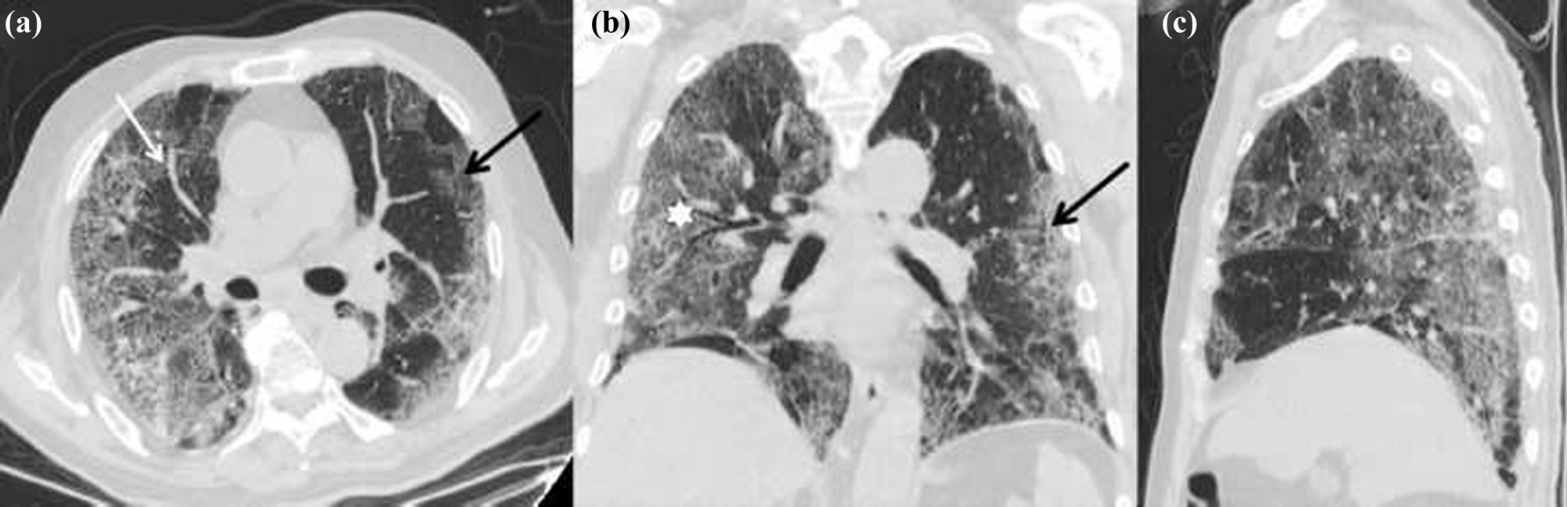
First CT scans of the patient (a: axial noncontrast CT image; b: coronal thin-section noncontrast CT image; and c: sagittal thin-section noncontrast CT image) showing large areas of GGO distributed mainly in subpleural (black arrows), thickened vascular shallow (white arrow), and bronchus shallow (white asterisk).
